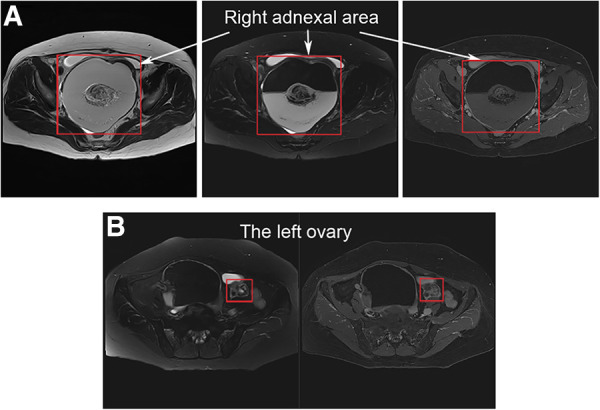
Magnetic resonance imaging of the abdominal cavity and pelvis: (A) a vast cystic-solid lesion in the right adnexal area; (B) the left ovary is enlarged with multiple vesicular changes.
